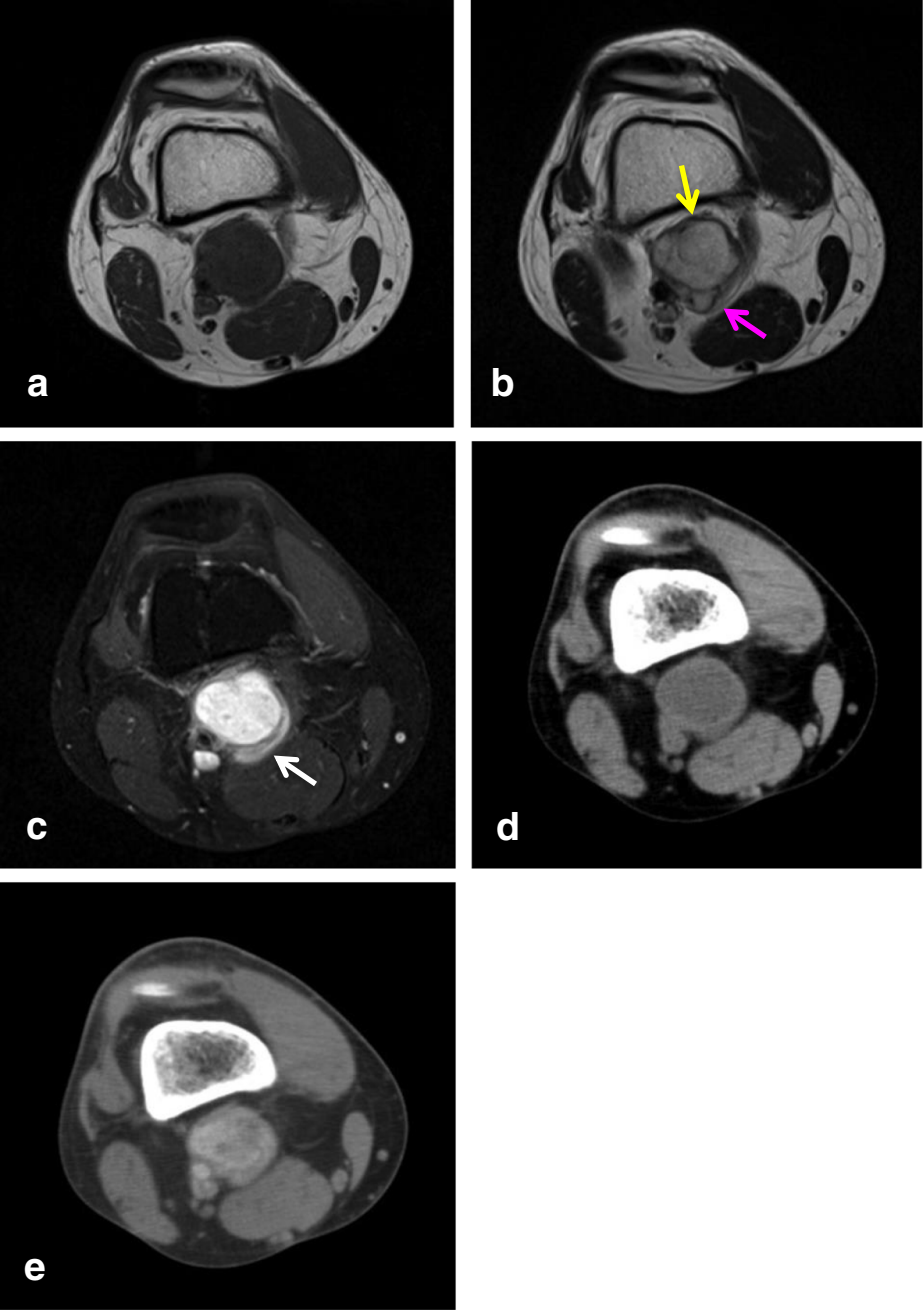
A 36-year-old man diagnosed with angiomatoid fibrous histiocytoma (case 5): (a) T1-weighted spin echo, (b) T2-weighted spin echo, and (c) contrast-enhanced MR images. (d) Non-enhanced and (e) enhanced CT images. A 32 × 36 × 45-mm asymptomatic mass is present in the popliteal lesion of right knee. The lesion is homogeneously isointense on T1 WI and presents with a multilocular area (pink arrow) and pseudocapsule (yellow arrow) on T2 WI. A contrast-enhanced MR image shows intratumoral and peritumoral (white arrow) enhancement. In addition, an enhanced CT image shows variegated enhancement
